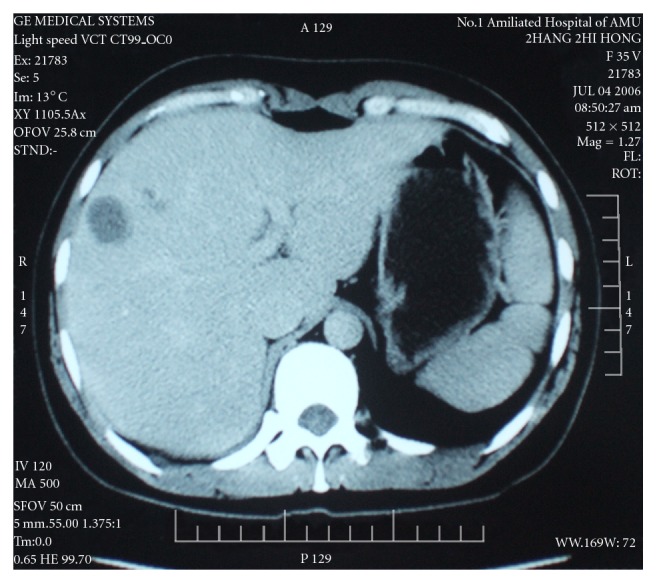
CT showed one low-density lesion under the right hepatic lobe capsule for case 1.
